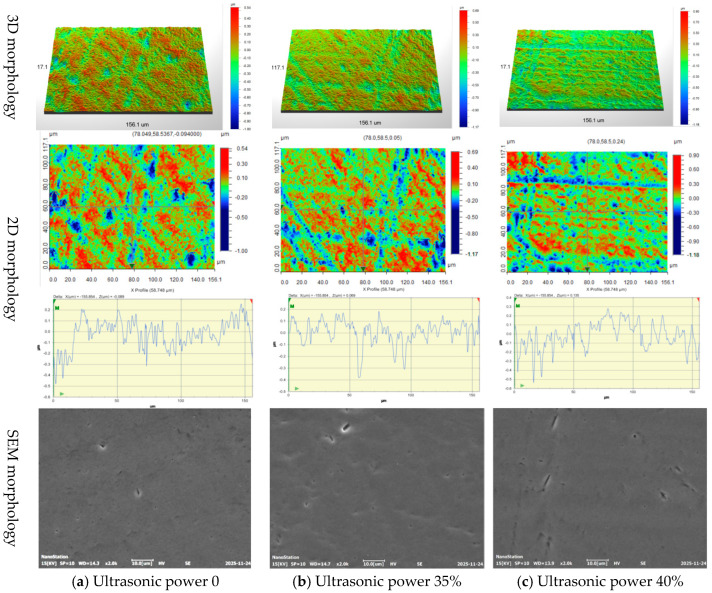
Surface morphology of monocrystalline silicon polished surface varies with the change in ultrasonic power. (a) Ultrasonic power 0 (b) Ultrasonic power 35% (c) Ultrasonic power 40%.
